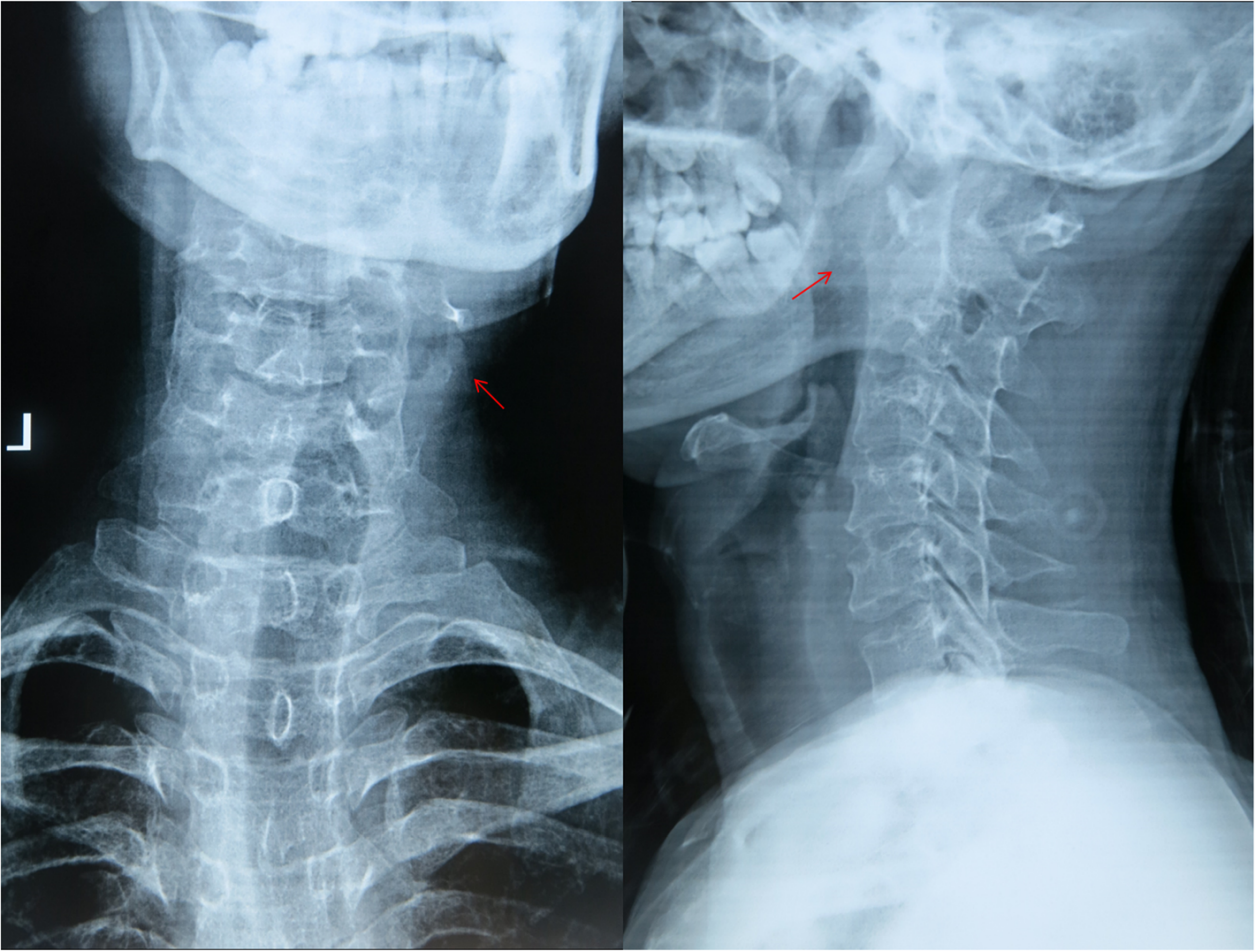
A male patient at 29 years old with atlantoaxial tuberculosis combined with neurological damage. The ASIA grading was C. Preoperative anteroposterior and lateral radiographs showed mild lateral curvature of the cervical spine and bone disorders of the upper cervical spine
